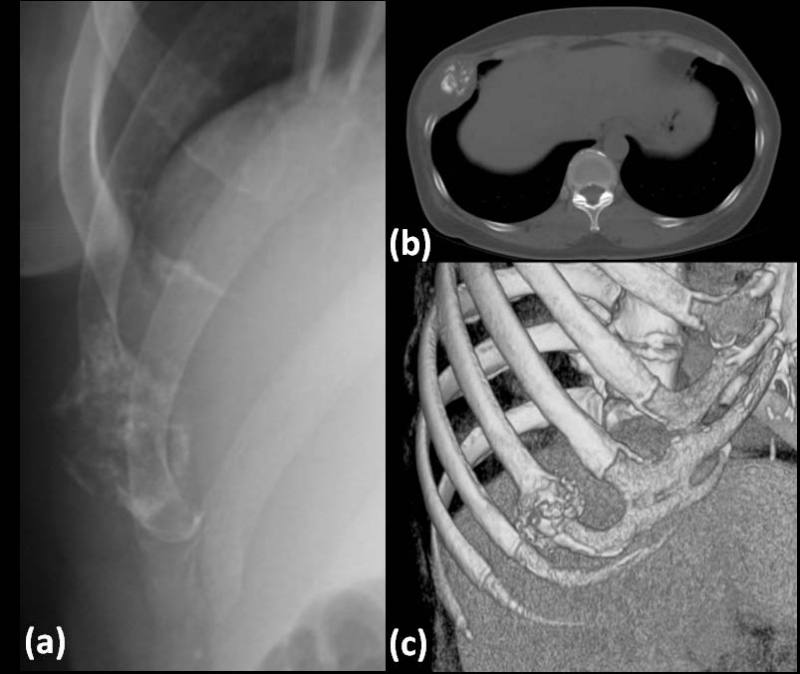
Preoperative radiological examinations*. *(a) X-ray showing a mass with coarse calcification located in the right eighth rib, expanding beyond the irregular cortex. (b) CT scan showing a low -density mass with coarse calcification along the right eighth rib; the mass arose at the bone-cartilage border. (c) 3D-CT scan showing destruction of bone and cartilage destruction, with expansive growth of the tumor at the right eighth rib*.
